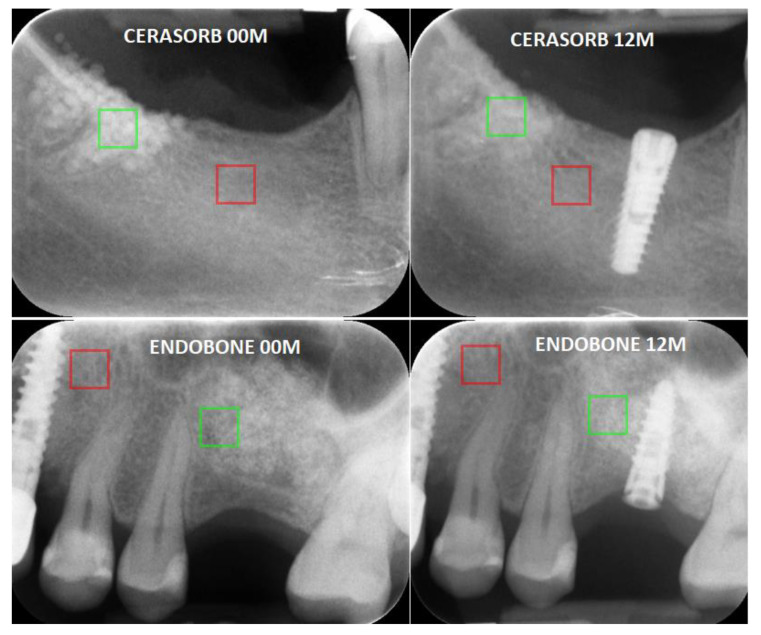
Examples of analysed intraoral radiographs (directly after implantation (00 M) and one year after surgery (12 M)). The exemplary regions of interest (ROI) are marked in green (material ROI) and red (bone ROI).
